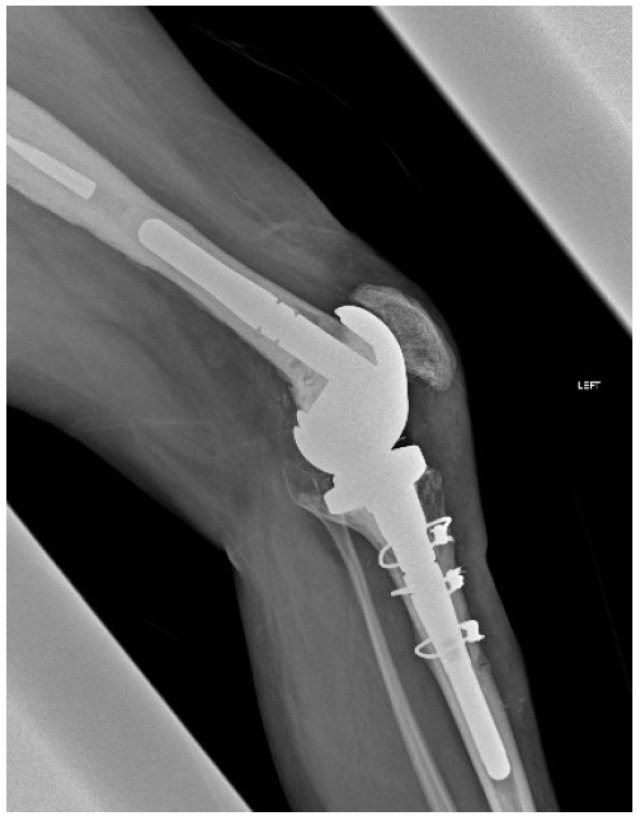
Lateral radiograph demonstrating bridging callus formation at the osteotomy site (Case 3).
